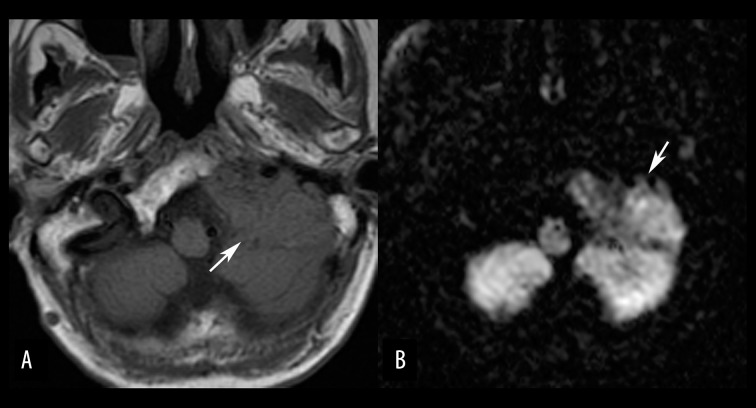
Multiple myeloma in a 76-year-old man. (A) Axial, non-contrast T1-weighted MR image shows a hypointense mass (arrow) destroying the left petrous bone and the left lower clivus, in contrast to normal hyperintense fatty bone marrow. (B) On an axial, diffusion-weighted image, the mass is hyperintense, equivalent to the brain tissue.
